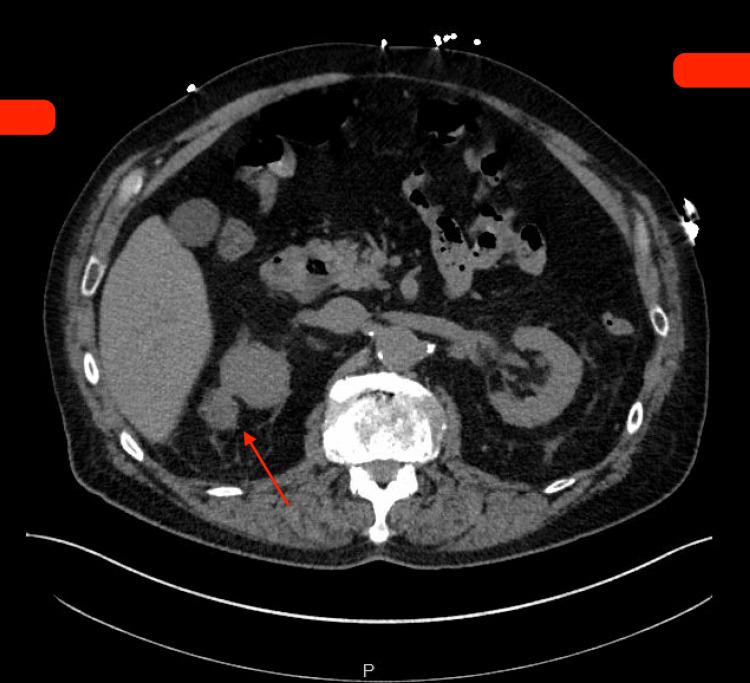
CT scan showing questionable adrenal masses that might represent metastasis.
